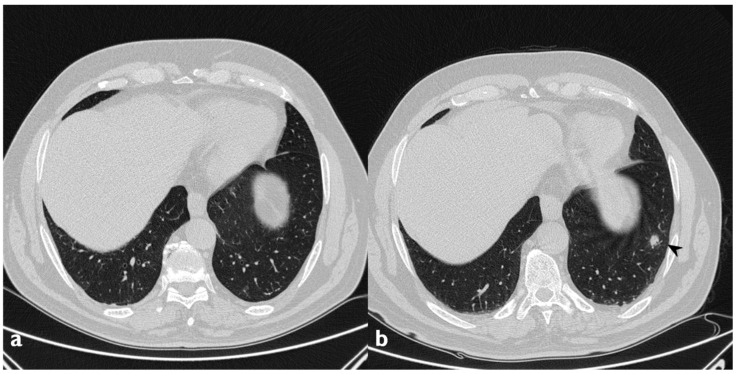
On the left (a): baseline HRCT. On the right (b): follow-up HRCT; a new nodule (arrowhead) appeared in the left lower lobe.
